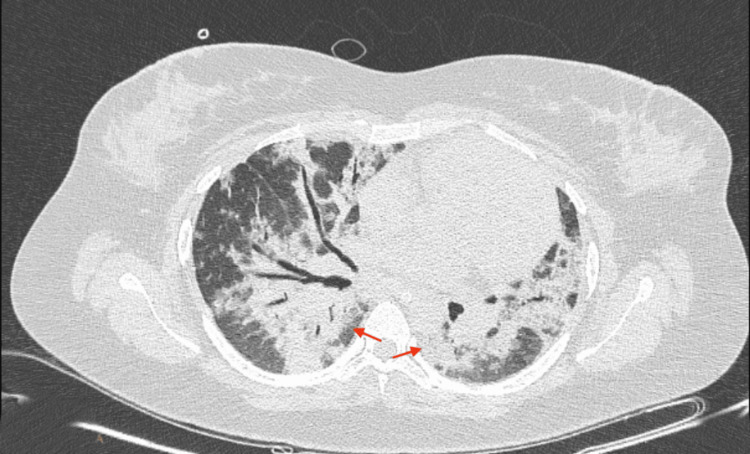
CT scan of the chest showing bilateral ground-glass opacities, consolidation, and interstitial thickening (arrows).
